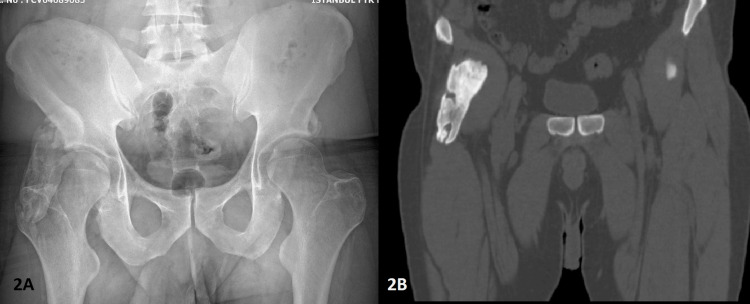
Radiographic and computed tomography findings of the lesion. (A) Plain radiograph showing heterotopic ossification. (B) Coronal computed tomography image demonstrating an ossified mass.
